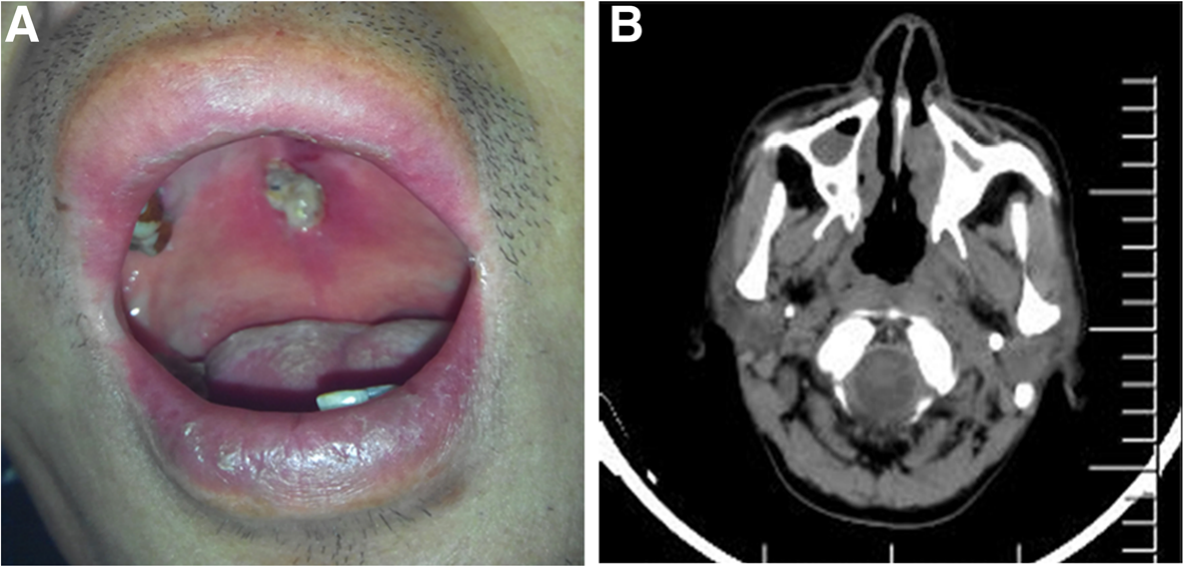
a An initial ulcer 1.5*0.5 cm in size with white purulent secretion and necrotic tissue on the surface could be seen on the palate. b Computed tomography scans revealed an occupied nasopharynx
